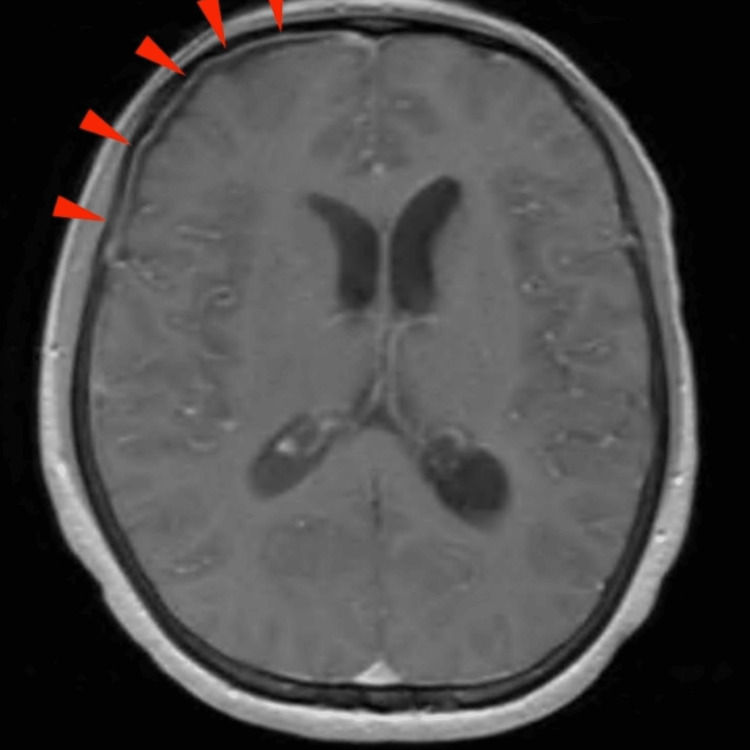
T1 post-contrast axial reconstruction of brain MRI demonstrating localized right frontal outer dural enhancement (red arrowheads) without leptomeningeal involvement.
